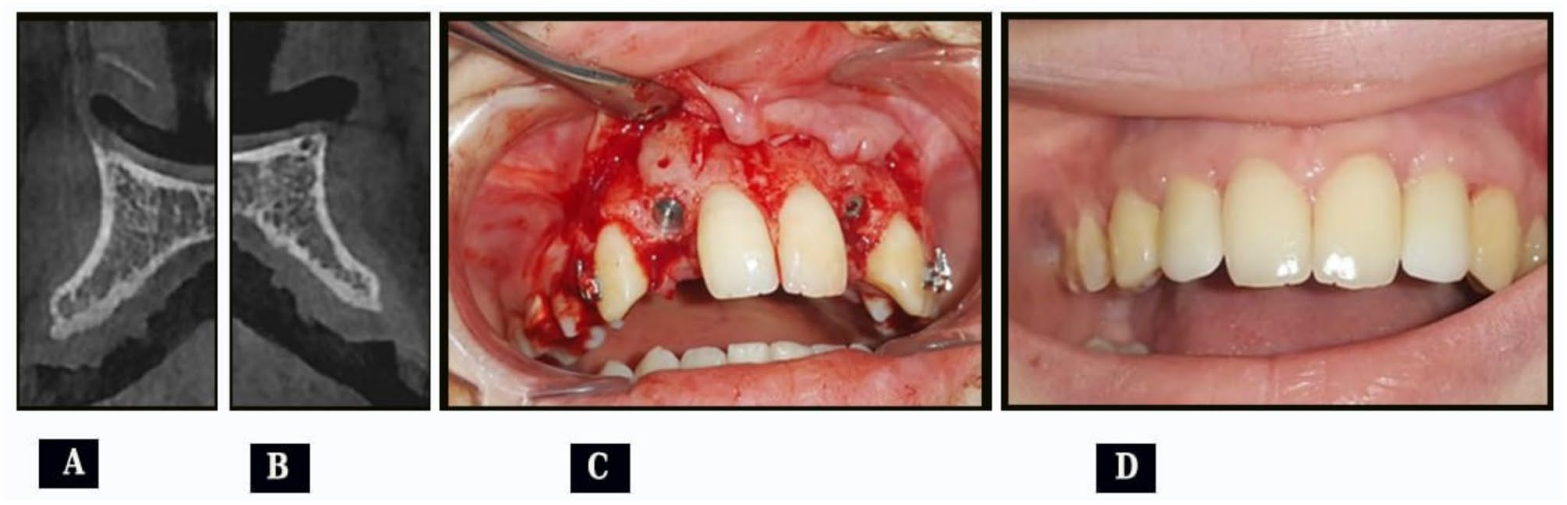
(case#6): A&B, Preoperative CBCT cross sectional view showing atrophied right (A) and left (B) alveolar ridges; (C), Removal of fixation screws and implant insertion; (D), Final restoration
